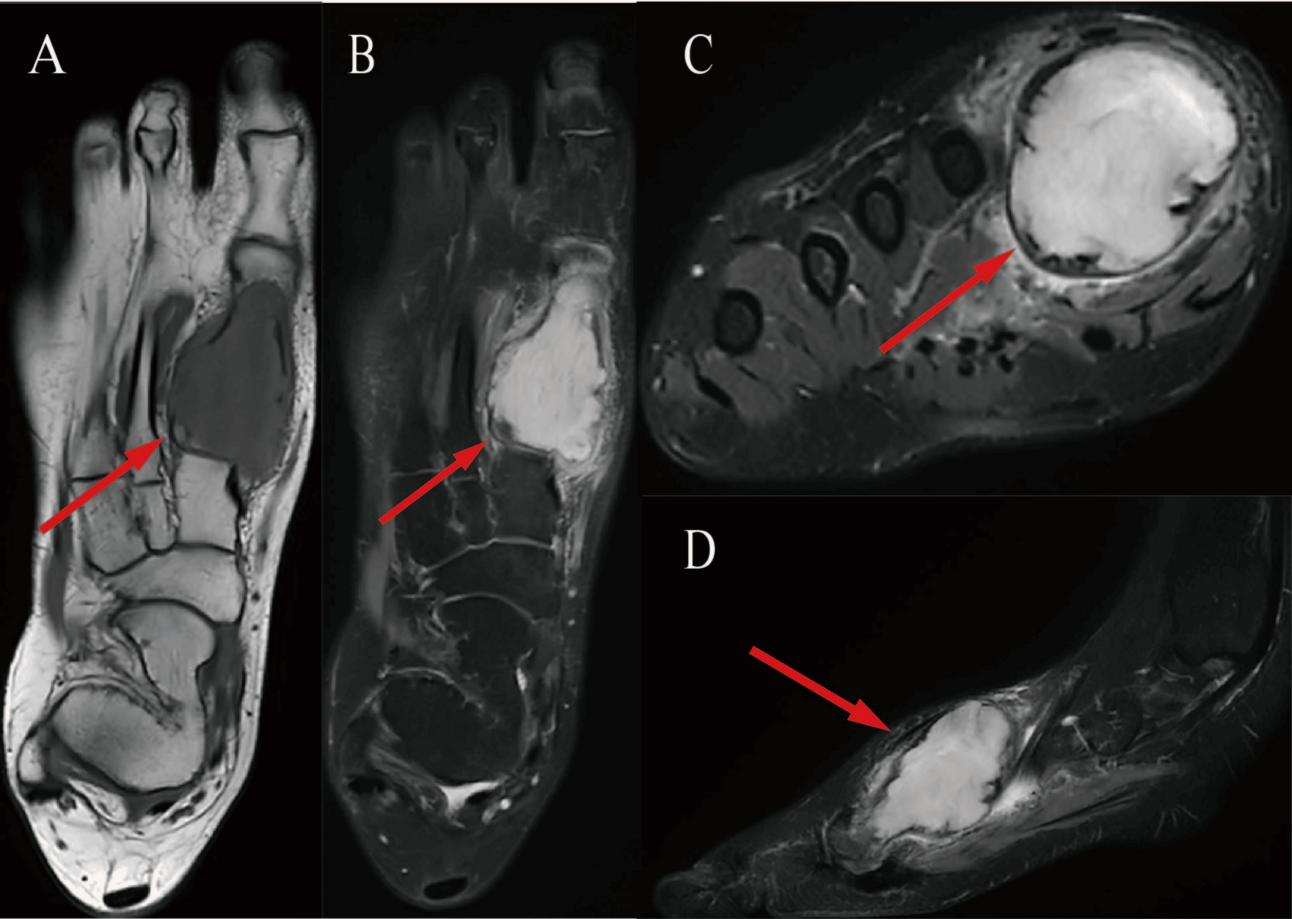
(A) Coronal T1-weigthted image, (B) coronal proton density weighted fat–saturated, (C) axial, and (D) sagittal T2-weighted fat–saturated MRI images (A) Coronal T1-weighted image, (B) coronal proton density weighted fat–saturated, (C) axial and (D) sagittal T2-weighted fat–saturated MRI images reveal a circumscribed neoplasm within the right first metatarsal bone, characterized by osseous expansion, cortical attenuation, and disruption of surrounding skeletal integrity. Signal characteristics include relative hypointensity on T1-weighted sequences and a pronounced hyperintense signal pattern on T2-weighted acquisitions.
